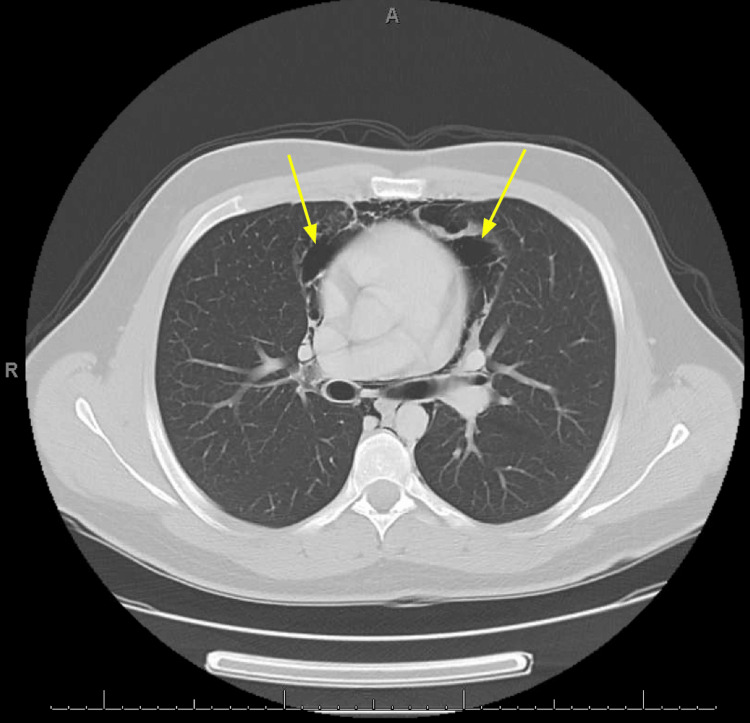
Computed tomography with contrast of the chest at the level of the inferior mediastinum showing air within the mediastinum
